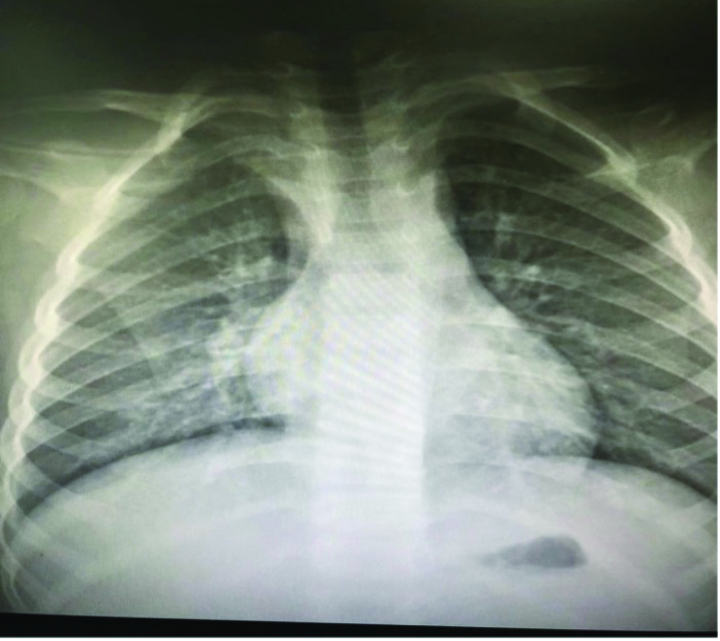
X-ray showing increased lung marking.
